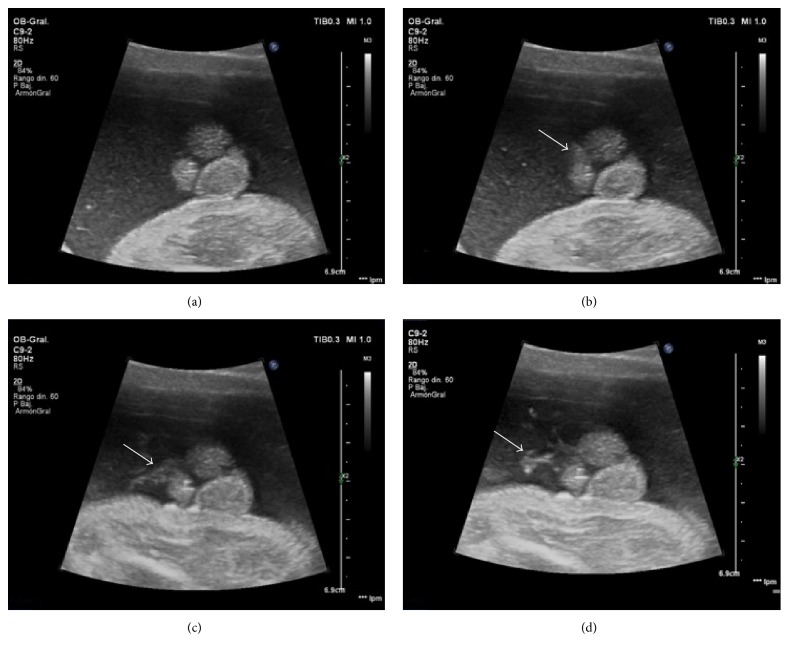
Consecutive sonographic images of the fetal micturition, achieved after external stimulation, which allow us to see the ventral urinary jet (arrows) near the penile base.
